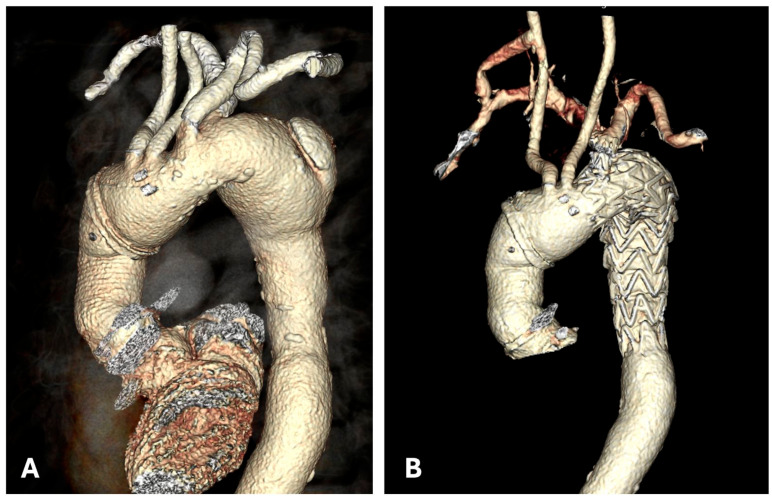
Volumetric rendering visualization via CTA: pre-procedure (A) and post-implantation of the prosthesis, carotid-subclavian bypass, and plug placement at the origin of ARSA (B).
